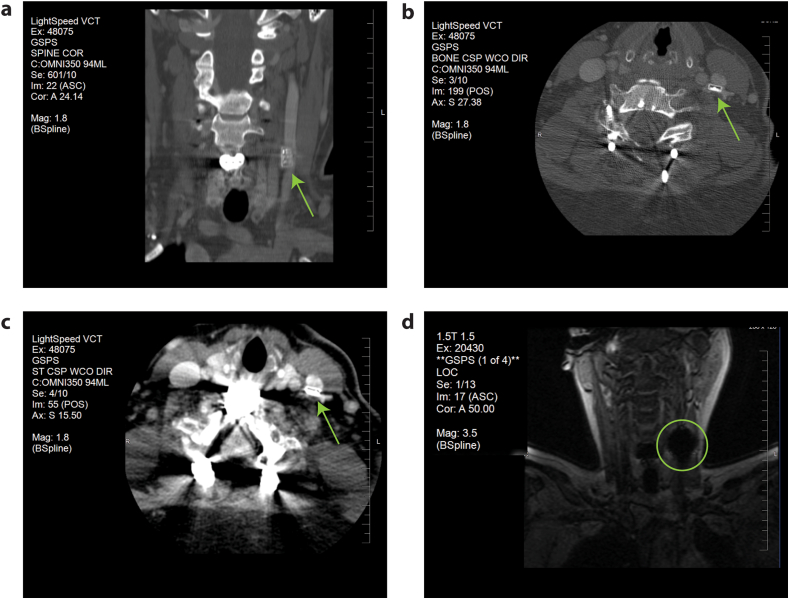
MEPS device is visible in CT imaging and occluded in MRI. The MEPS device is visible in three CT images (A, B, C), with key scan parameters labeled for each. The CT images, using a slice thickness ranging from 2.4 to 5.15 ​mm, provide clear anatomical localization of the device (indicated by green arrows). Magnification (1.8×) and contrast agent (Omnipaque 350, 94 ​mL) enhance visibility in both bone and soft tissue views. The MRI image (D) shows the expected artifact (marked with green circle) caused by the device, which occludes its visibility. However, the size of the artifact does not interfere with the usefulness of 1.5T MRI imaging for routine diagnostic purposes, allowing evaluation of surrounding structures. This combination of imaging modalities demonstrates the device's compatibility with both CT and MRI for routine post-operative care.
